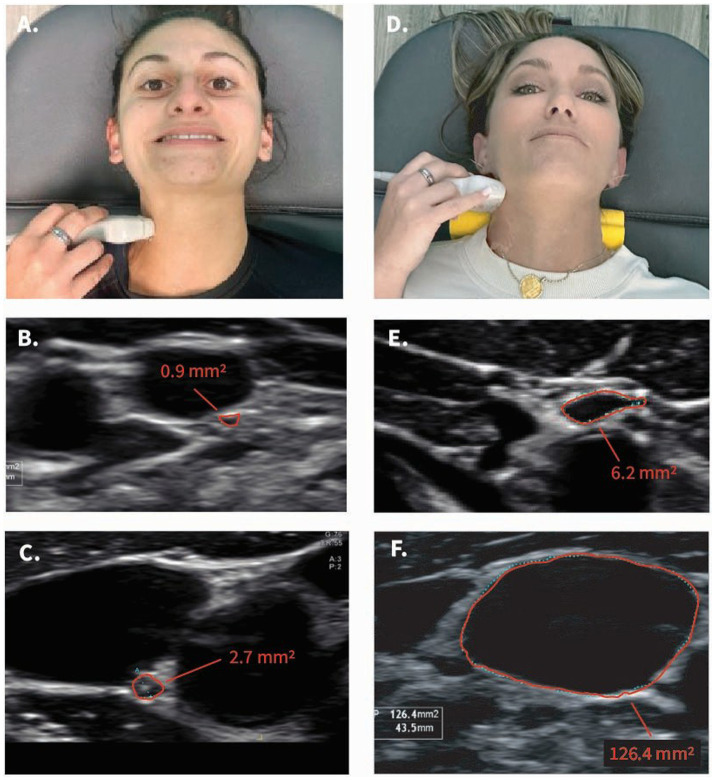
Ultrasound measurement of internal jugular vein (IJV) and vagus nerve as part of full diagnostic workup. (A) Probe placement of vagus nerve at C4-C5. (B) Abnormal vagus nerve at C4-C5, <1 mm. (C) Normal vagus nerve at C4-C5, >2 mm. (D) Probe placement of IJV at the C1 level lying on a Denneroll^®^. (E) Abnormal IJV, <40 mm. (F) Normal IJV, >90 mm.
